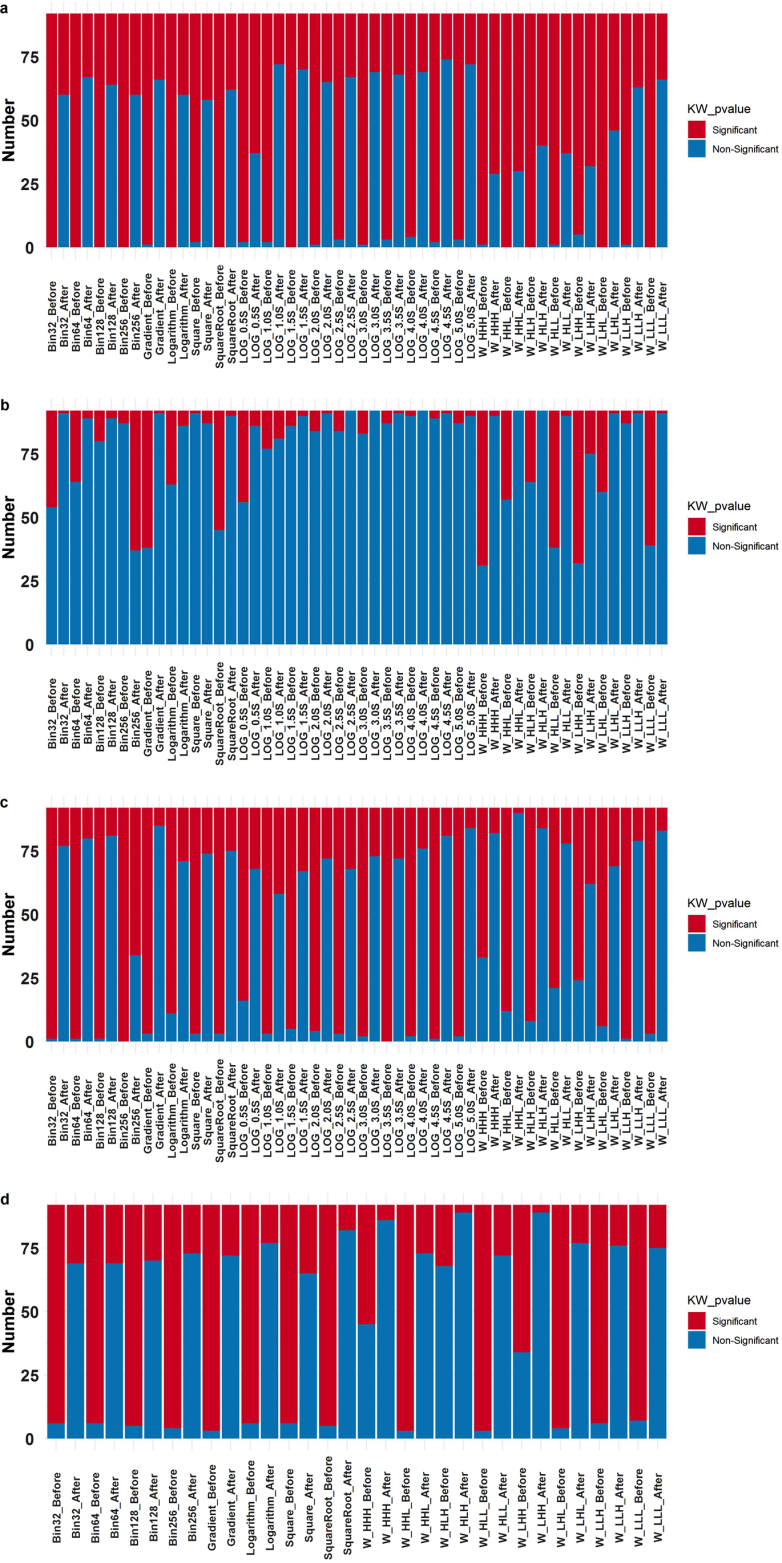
Results of Kruskal-Wallis’s (KW) test before and after ComBat harmonization, over various image pre-processing techniques for MR images acquired on: a four scanners, b three times test, c various flip angles, and d various inversion recoveries. LOG, Laplacian of Gaussian (LOG); S, sigma; W, wavelet; L, low-pass filter; H, high-pass filter
